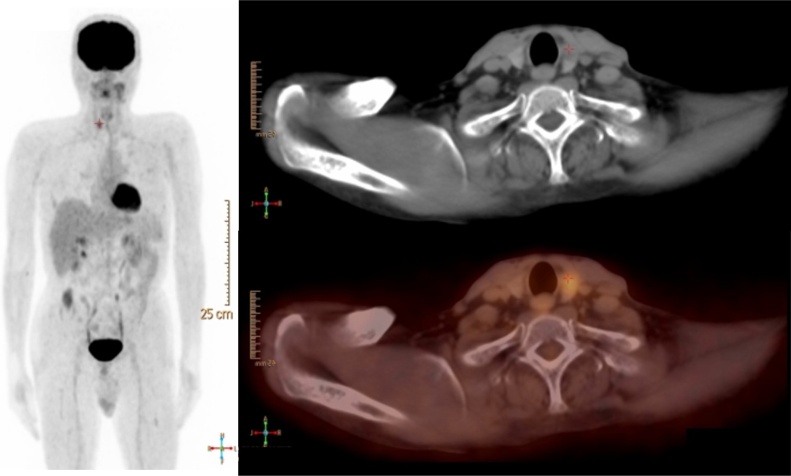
52 years old male with Wegner’s granulomatosis and left buccal squamous cell carcinoma. F-18 FDG PET/CT showed a hypermetabolic hypodense right thyroid lobe nodule with SUVmax of 5.7 and CT HU of 64. FNA cytology revealed papillary thyroid cancer.
